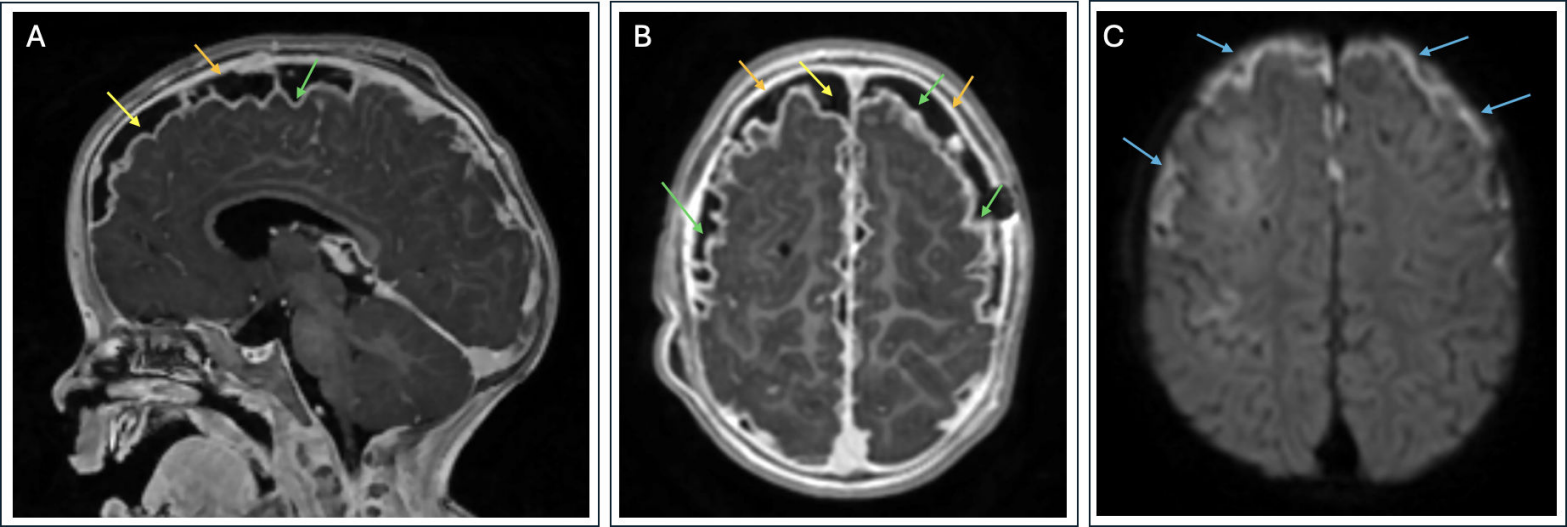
Brain MRI with and without contrast. Image (A) displays a sagittal T1-weighted post-contrast image; image (B) is an axial T1-weighted post-contrast image; and image (C) is an axial diffusion-weighted image. There is diffuse leptomeningeal (green arrows) and pachymeningeal (orange arrows) enhancement associated with bilateral hypoenhancing fluid collections along the cerebral convexities (yellow arrows) displaying restricted diffusion (blue arrows). This is consistent with meningitis complicated by subarachnoid empyema.
